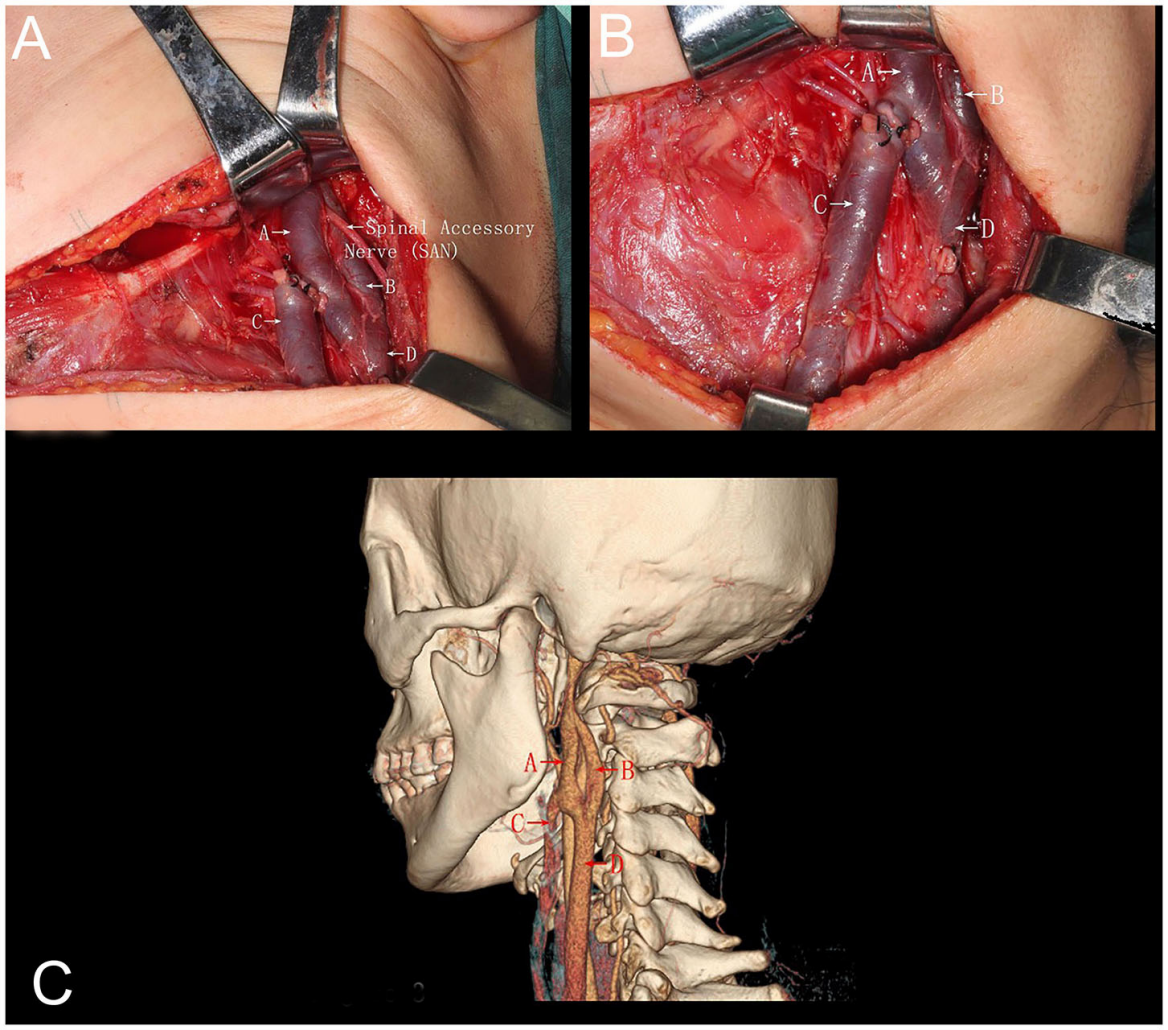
(A) Intraoperative picture demonstrating a left-side neck dissection. The internal jugular vein divided into two branches anterior (A) and posterior (B) from its origin at the jugular foramen. Both branches run downwards parallel to each other for ~4 cm in length and fused back together (D), demonstrating a window-like opening between the two branches. The anterior branch of the internal jugular vein received blood from an anterior tributary vein (C). Additionally, the spinal accessory nerve transversed this fenestration (A, anterior branch internal jugular vein; B, posterior branch internal jugular vein; C, anterior tributary vein; SAN, spinal accessory nerve; D, internal jugular fusion after fenestration). (B) Intraoperative picture of a left-side neck dissection, demonstrating the course of the anterior tributary vein (C) running down on the anterolateral region and deep into the platysma (A, anterior branch internal jugular vein; B, posterior branch internal jugular vein; C, anterior tributary vein; SAN, spinal accessory nerve; D, internal jugular fusion after fenestration. (C) Three-dimensional computed tomographic angiography corroborating the intraoperative findings (A, anterior branch internal jugular vein; B, posterior branch internal jugular vein; C, anterior tributary vein; SAN, spinal accessory nerve; D, internal jugular fusion after fenestration).
